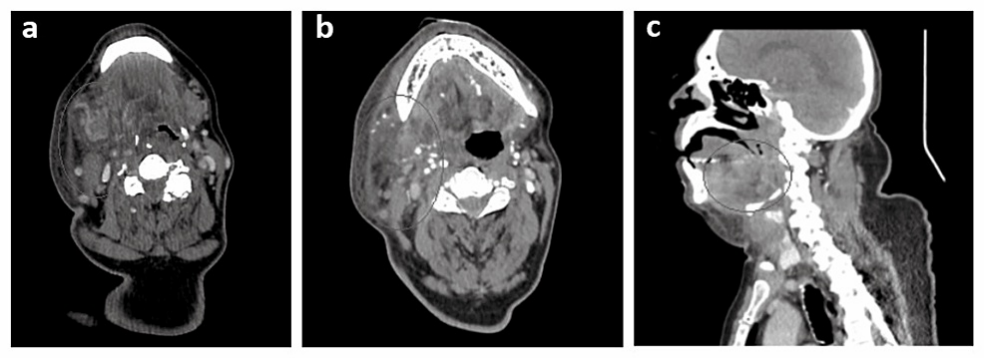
The patient’s computed tomography scan of the head and neck. (A) An axial image indicating asymmetrical right parapharyngeal enlargement causing effacement of the adjacent pharyngeal space (encircled in black). (B) An axial image indicating right submandibular and sublingual soft tissue swelling (encircled in black). (C) A sagittal image indicating significant submental swelling (encircled in black).
